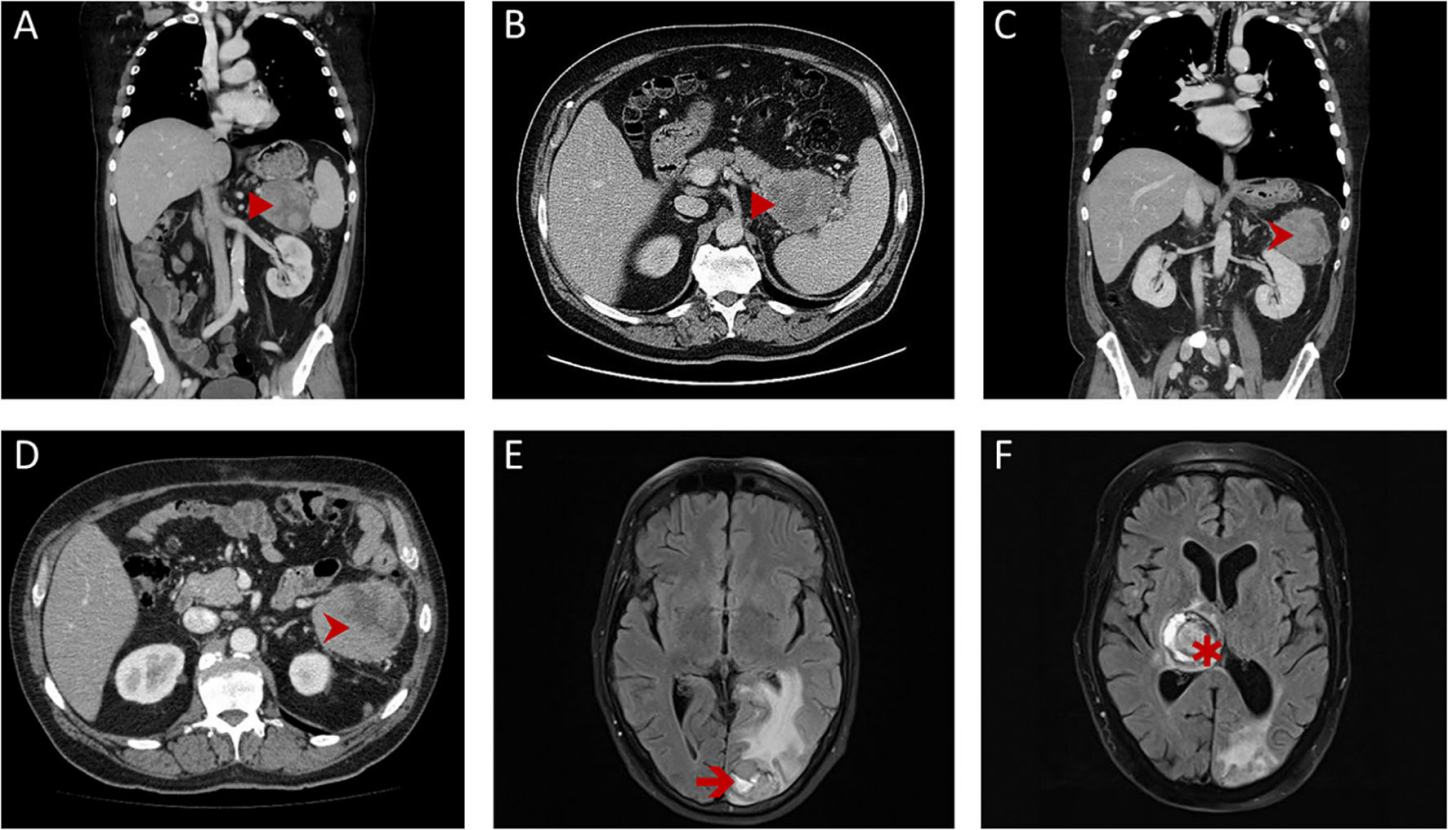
Radiological findings. First contrast-enhanced computed tomography (CEPT; A, B) of the thorax and abdomen revealed a large tumor (►) of the pancreatic tail, close to the splenic hilum with a pseudo-encapsulated appearance. Follow-up CEPT (C, D) showed isolated local recurrence (➤) with similar features in the left-upper abdomen and the retroperitoneum. Repeated magnetic resonance imaging scans of the brain showed a left occipital metastasis (➔) shortly after resection for local recurrence (E) and a rapidly progressing metastasis of the thalamus region (✱) over the course of palliative systemic treatment (F).
